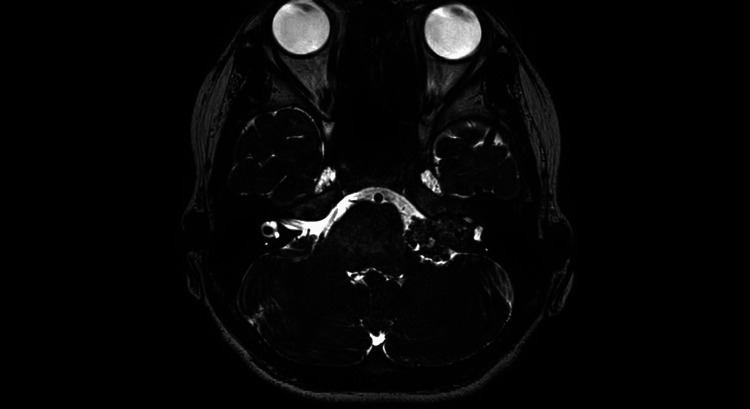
Preoperative MRI heavy T2-weighted image It mildly displaced the brainstem and cerebellum and filled the internal auditory canal.
